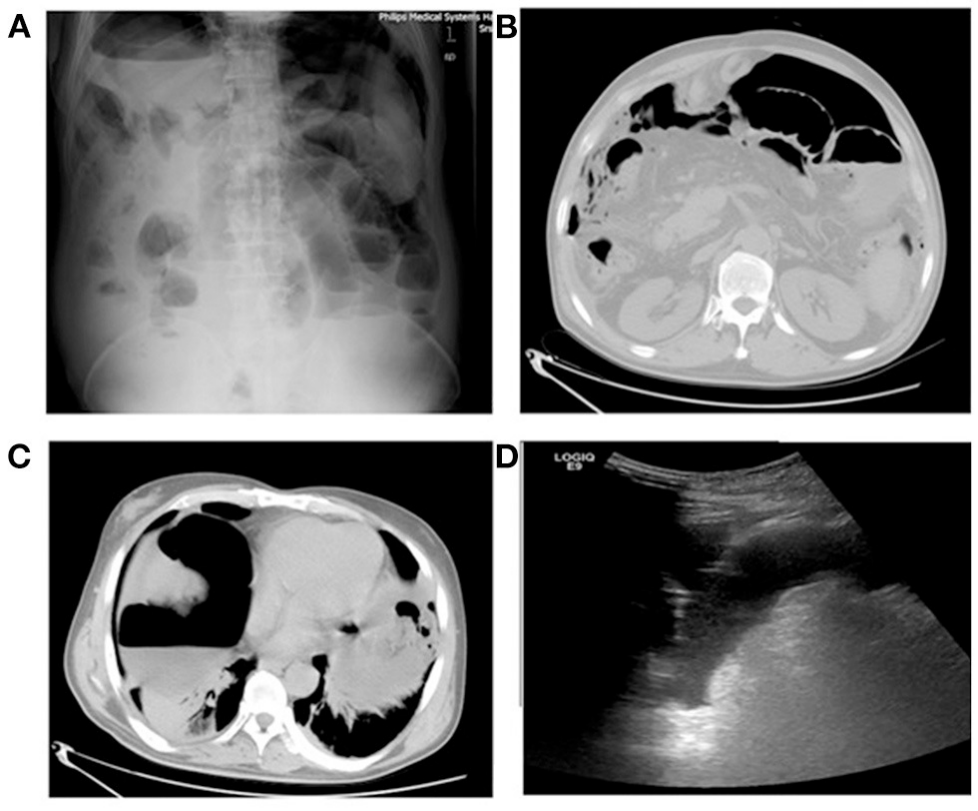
Patient number 1: male, 64 years, hepatocellular carcinoma (S8 segment). Ultrasound examination showed pelvic effusion and poor sound transmission on 4 days after microwave ablation. CT radiography of the abdomen (standing) showed free intraperitoneal air. (A) Radiography of the abdomen (standing) 6 days after microwave ablation. (B) CT 6 days after microwave ablation. (C) CT 6 days after microwave ablation. (D) Ultrasound of the abdomen 4 days after microwave ablation.
